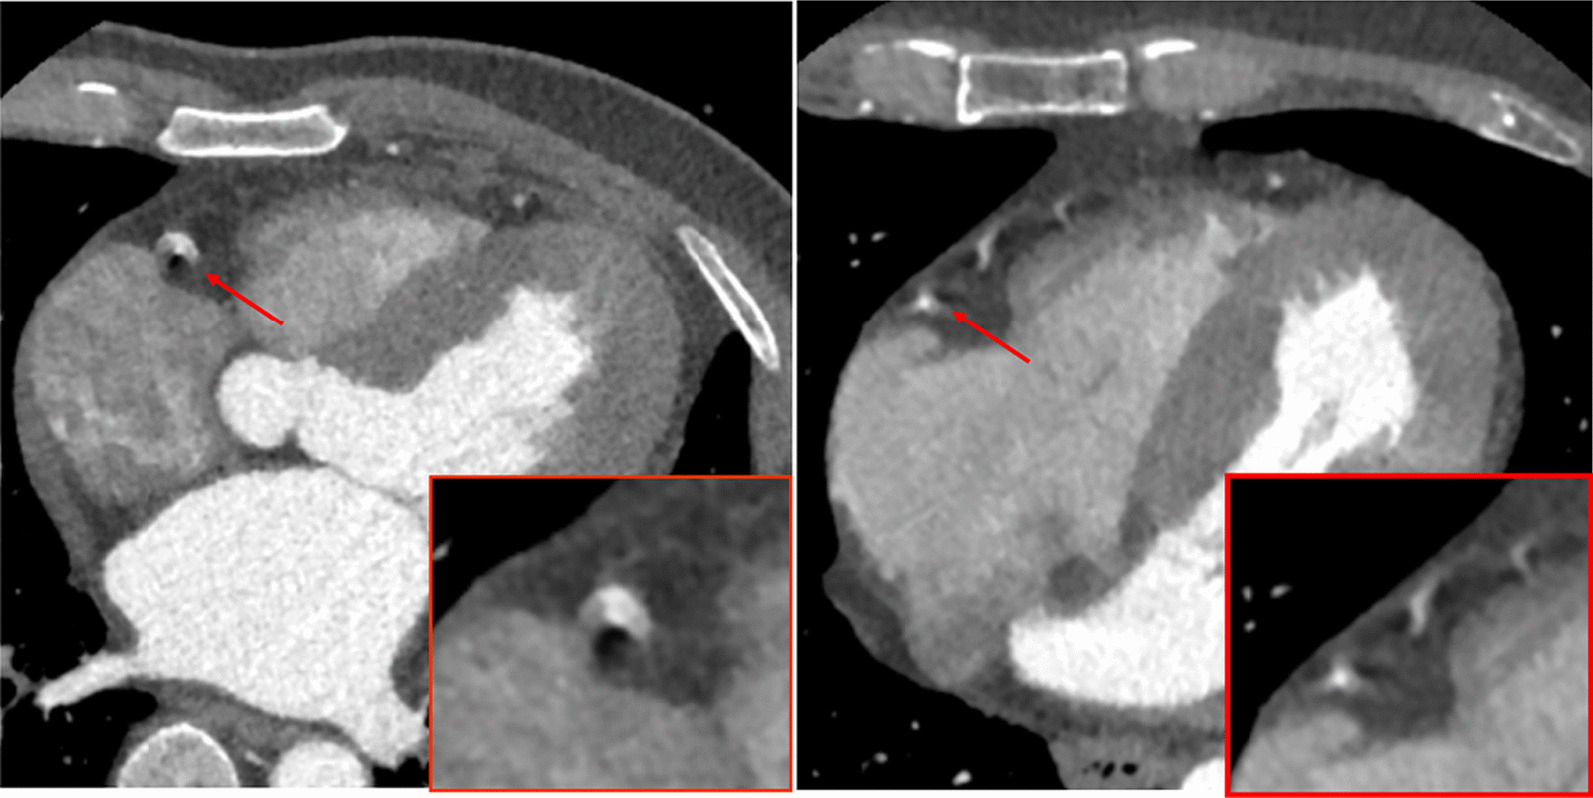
Cardiac motion leads to differently shaped artefacts in CT scans
